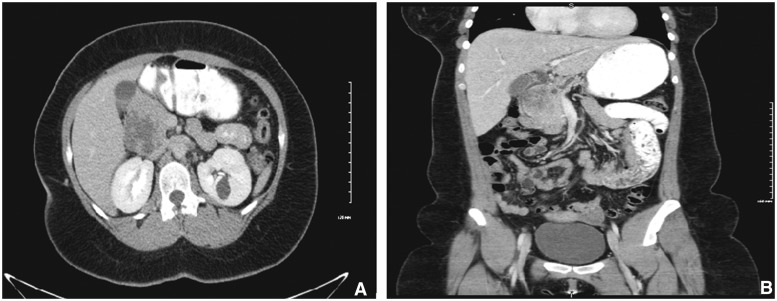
CT Abdomen and Pelvis (A) with and (B) without contrast showing pancreatic mass.
